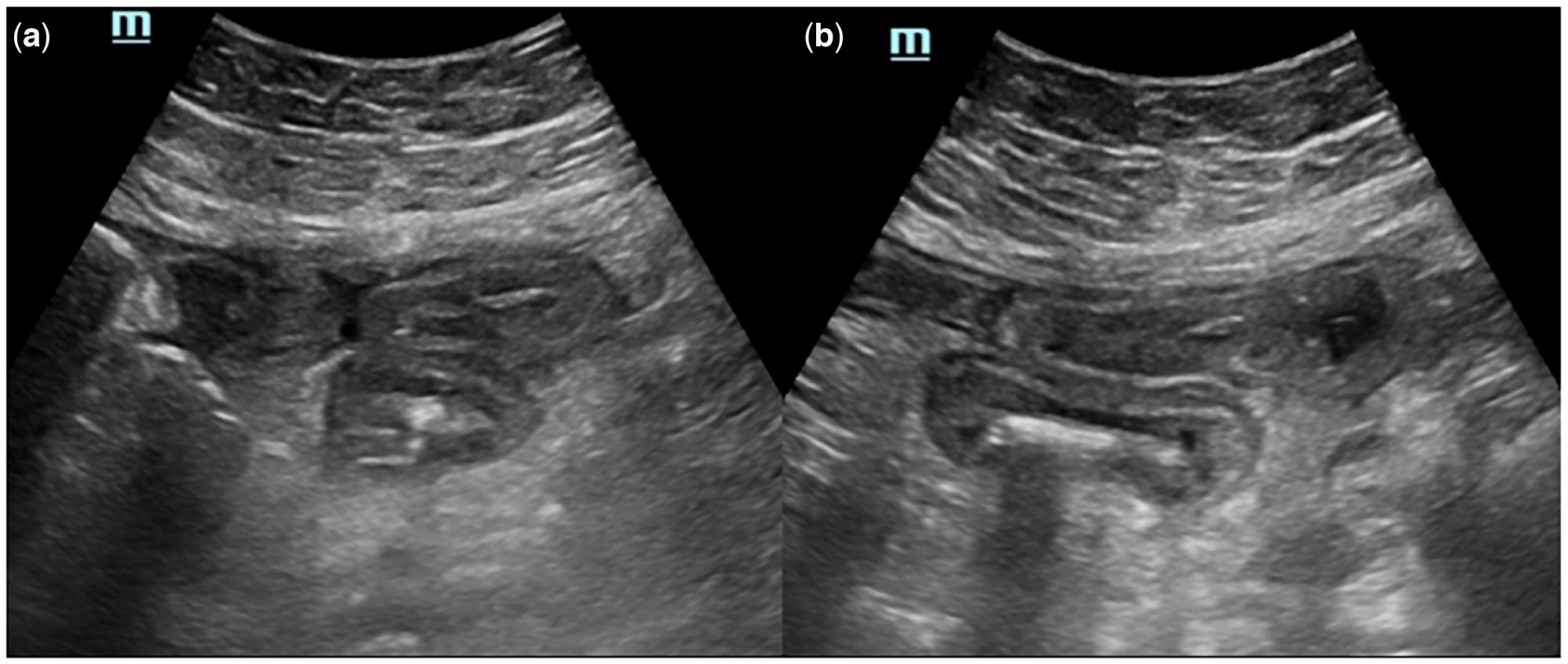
Abdominal ultrasonography demonstrates diffuse and symmetrical wall thickening of the small bowel loops in a long section of the abdomen at midline level (A, B).
